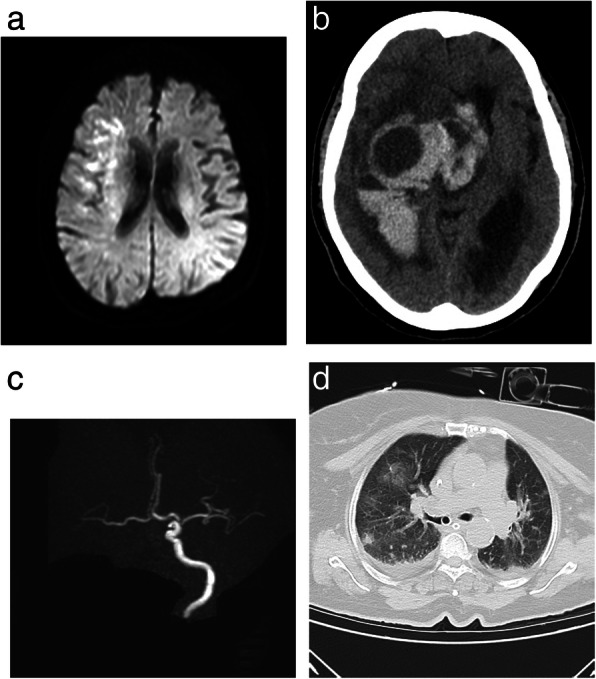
Patient 8, a MRI DWI with right external watershed infarction, b complete occlusion of right internal carotid artery, c follow-up CT brain after sudden deterioration on day 12 showing hemorrhagic transformation, interventricular extension, and midline shift, d CT chest showed 2 small areas of ground glass appearance, mild right and minimal left-sided pleural effusion, low to intermediate left-sided pleural effusion
